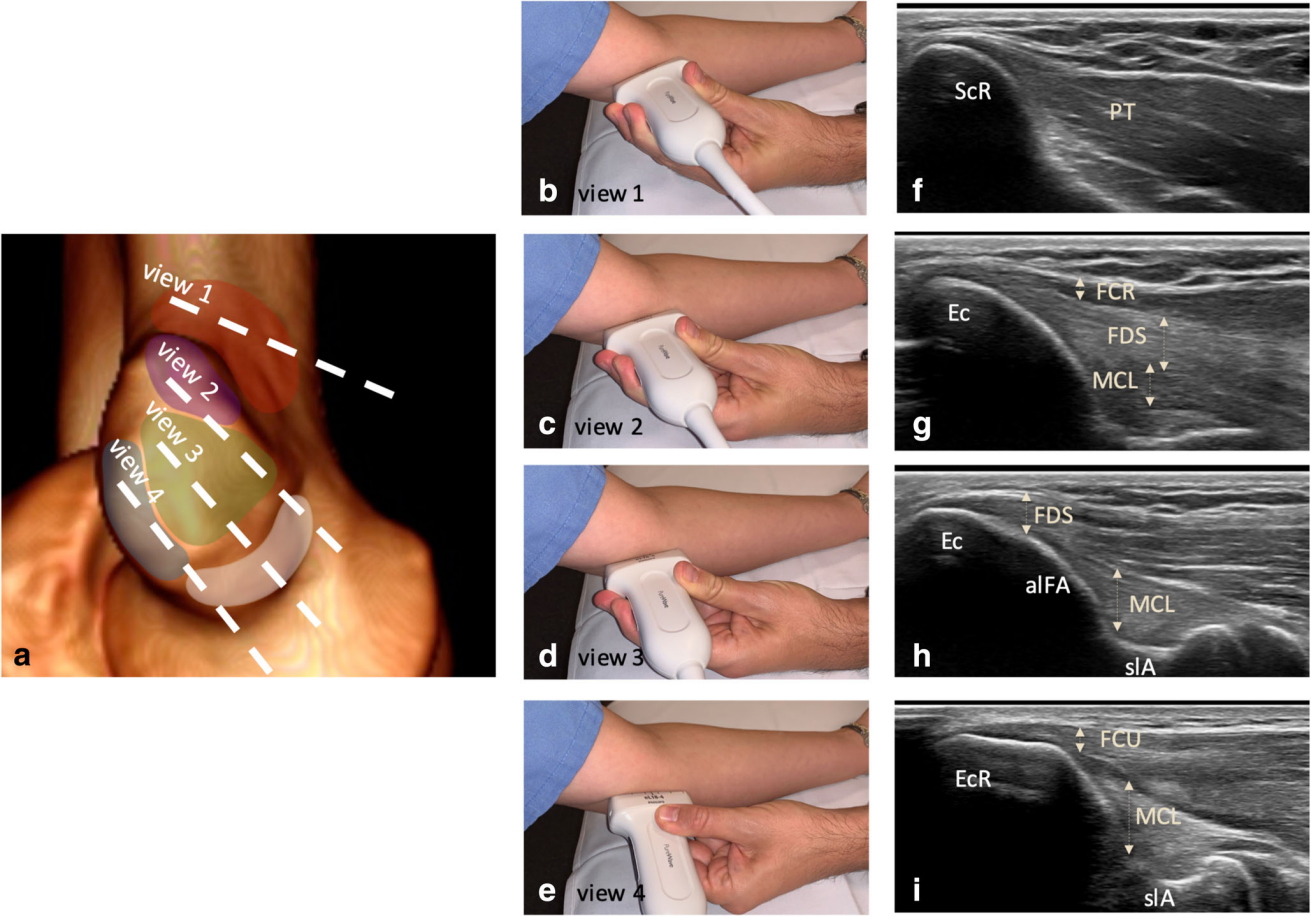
Ultrasonographic assessment technique for the medial epicondyle with position of the different ultrasonographic views on the lateral epicondyle shown on a CT with surface rendering (a), on photographs of a volunteer (b–e), with corresponding ultrasonographic images (f–i): View 1: supracondylar ridge (easily identified in the short-axis plane of the distal humerus): the muscular attachment of the pronator teres onto the supracondylar ridge is identified. View 2: anterosuperior aspect of epicondyle (slightly moving the probe distally to the epicondyle from View 1, at its anterior aspect): from superficial to deep, three structures can be differentiated by the presence of echogenic lines/difference of echogenicity: the FCR tendon, the FDS tendon and the MCL. View 3: intermediate view (between views 2 and 4): from superficial to deep, three structures can be differentiated by the presence of echogenic lines/difference of echogenicity: the palmaris longus tendon (inconsistent; not visible here), the FDS tendon and the MCL. View 4: epicondylar ridge: from surface to deep, the FCU tendon and the MCL can be easily identified. FCR: flexor carpi radialis, FDS: flexor digitorum superficialis, PL: palmaris longus, MCL: medial collateral ligamentous complex, FCU: flexor carpi ulnaris, ScR : supracondylar ridge, Ec : epicondyle, EcR : epicondylar ridge
